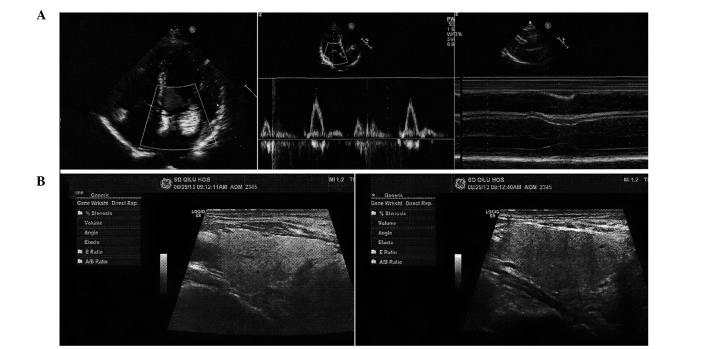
(A) An ultrasonic cardiogram revealed enlargement of the right atrium, widening of the ascending aorta, moderate mitral valve regurgitation, light medium tricuspid regurgitation and mild pulmonary valve regurgitation. (B) Ultrasound scans show that the thyroid gland was normal in size; however, a cyst (0.15 × 0.13 cm) was observed in the left lower pole.
